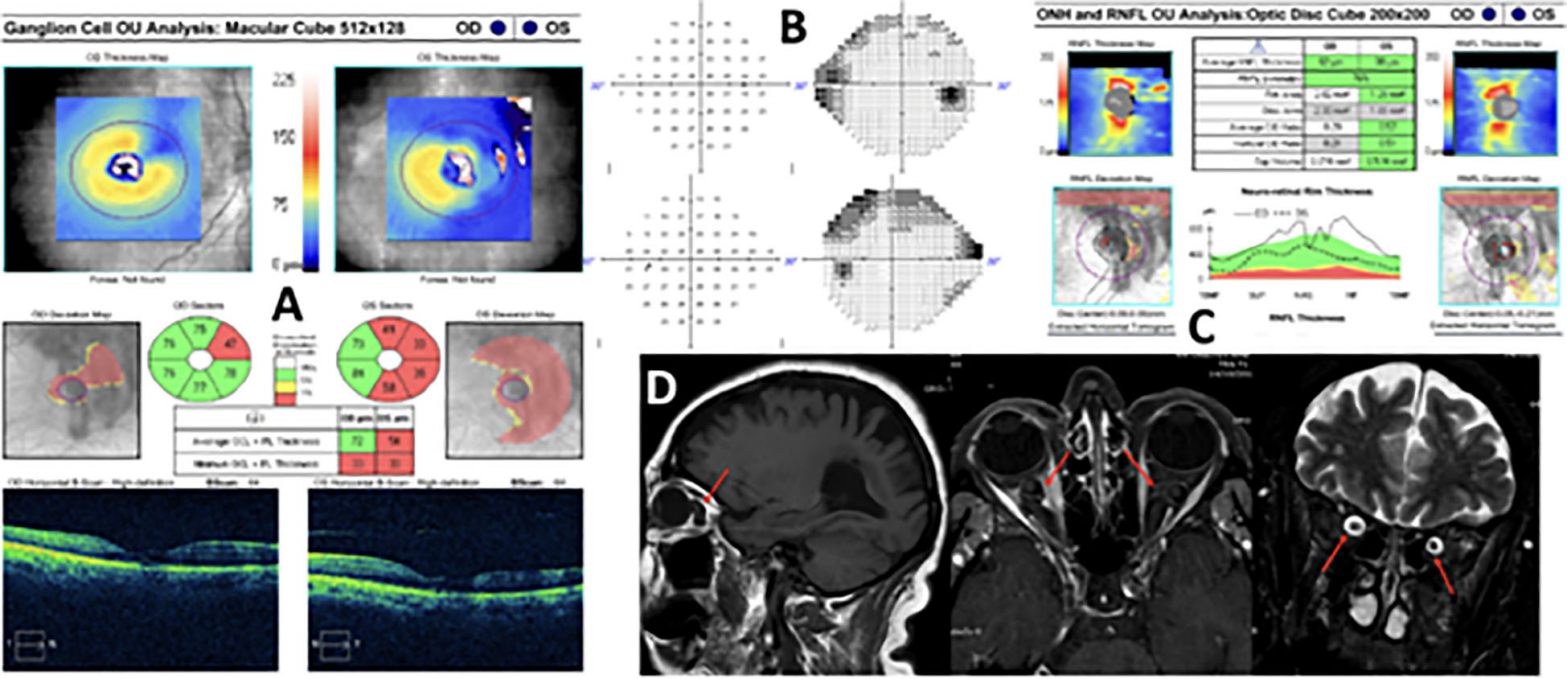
Case 3 (images from 2023—10 years of follow-up showing no progression in the VF, OCTs, or MRI since the initial diagnosis). (A) Ganglion cell layer sectors of both eyes. Left eye is artifacted. (B) 24-2 Visual field of both eyes with unspecific nasal and superior defects. (C) ONH OCT scan shows no optic disc edema, with normal RNFL. (D) MRI sagittal (left, T1-weighted), axial (middle, T1-weighted), and frontal (right, T2-weighted) views show bilateral optic nerve sheath dilatation and optic nerve tortuosity (red arrows).
